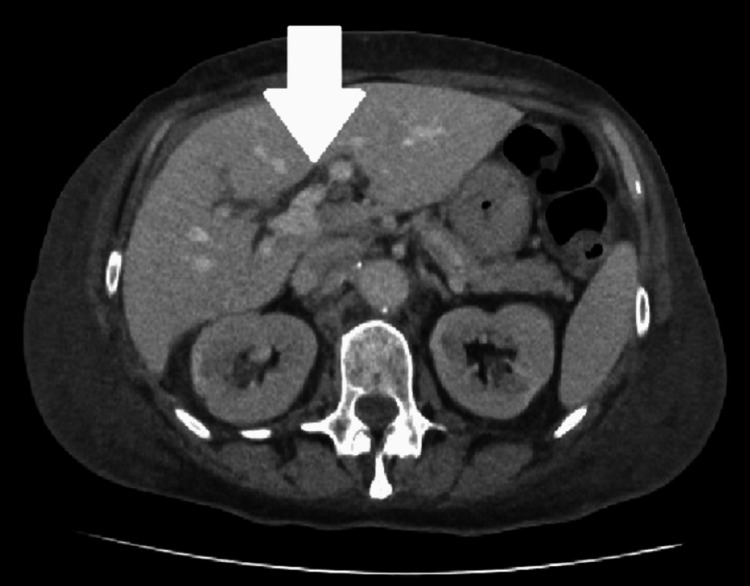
Contrast-Enhanced CT Abdomen Showing Periportal Oedema Axial CT image of the abdomen demonstrating periportal oedema (white arrow), with surrounding anasarca. Small-volume ascites and soft tissue oedema are also present, consistent with fluid overload secondary to nephrotic syndrome.
